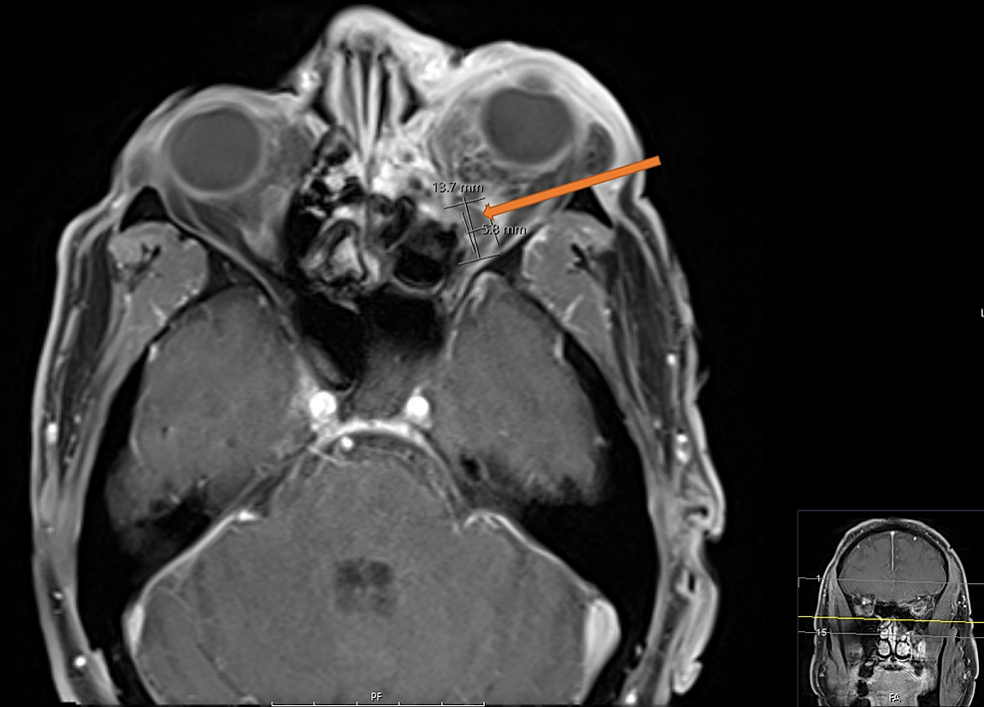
MRI of the face. Impression: Proptotic left globe with left intraorbital cellulitis. There is evidence of a 0.5 x 1.3 x 1.3 cm (transverse, anteroposterior, and craniocaudal dimension) subperiosteal abscess (orange arrow) within the inferomedial aspect of the left orbit secondary to contiguous extension from paranasal sinus disease. There is associated left cavernous sinus thrombosis.
